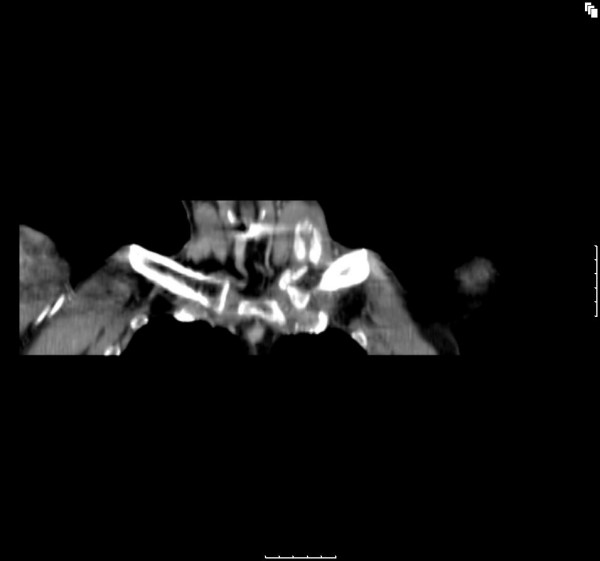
Reformatted coronal CT image showing a fracture of the clavicle with an osseous lesion within the sternocleidomastoid muscle (day 8).
